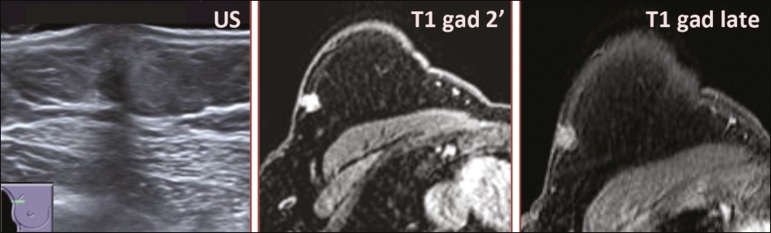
Ultrasound and MRI in a 59-year-old woman (case 4) presenting with a nodule in the upper outer quadrant of the right breast. The ultrasound shows that the mass is subcutaneous, a feature that has been recognized as a clue to the diagnosis of GCT. In gadolinium contrast-enhanced T1-weighted fat-saturated images, the nodule enhances avidly and shows washout. Note the fine spiculated margins. The morphologic features associated with this (type 3) kinetic curve would raise the suspicion of a malignant lesion. Pathology confirmed the diagnosis of GCT.
